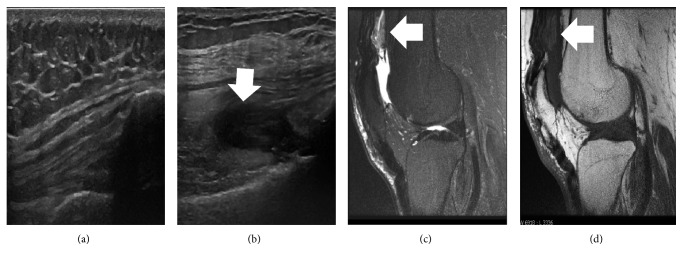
Point-of-care musculoskeletal ultrasound and magnetic resonance imaging of suprapatellar recesses in the knees. Longitudinal MSKUS image (a) of the unaffected knee was compared to the affected knee (b), which showed semicompressible hypoechoic contents (white arrow) in the suprapatellar recess. T2-weighted (c) and T1-weighted (d) MRI showed hyperintense (white arrows), corresponding to blood clots. MSKUS, musculoskeletal ultrasound; MRI, magnetic resonance imaging.
